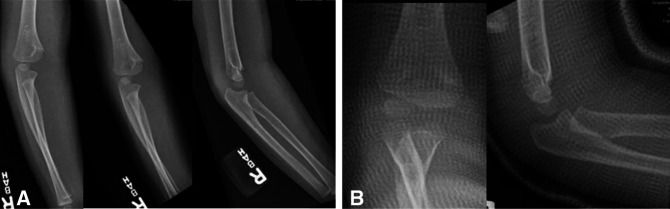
A, Radiographs showing the three views of an elbow fracture referred for surgical management because the capitellar ossification center appears angulated posterior to the anterior humeral line, that is, a Gartland Type II. B, Recognizing that this is not a true lateral, because the patient was likely unable to tolerate a lateral due to discomfort, the patient was immobilized for comfort, and radiographs repeated with the patient supine and the shoulder externally rotated, with the beam directed from medial to lateral. This provides the perfect lateral, with the ‘hourglass’ sign, and demonstrates this to be a more anatomic Gartland Type I, precluding the need for reduction or surgery.
